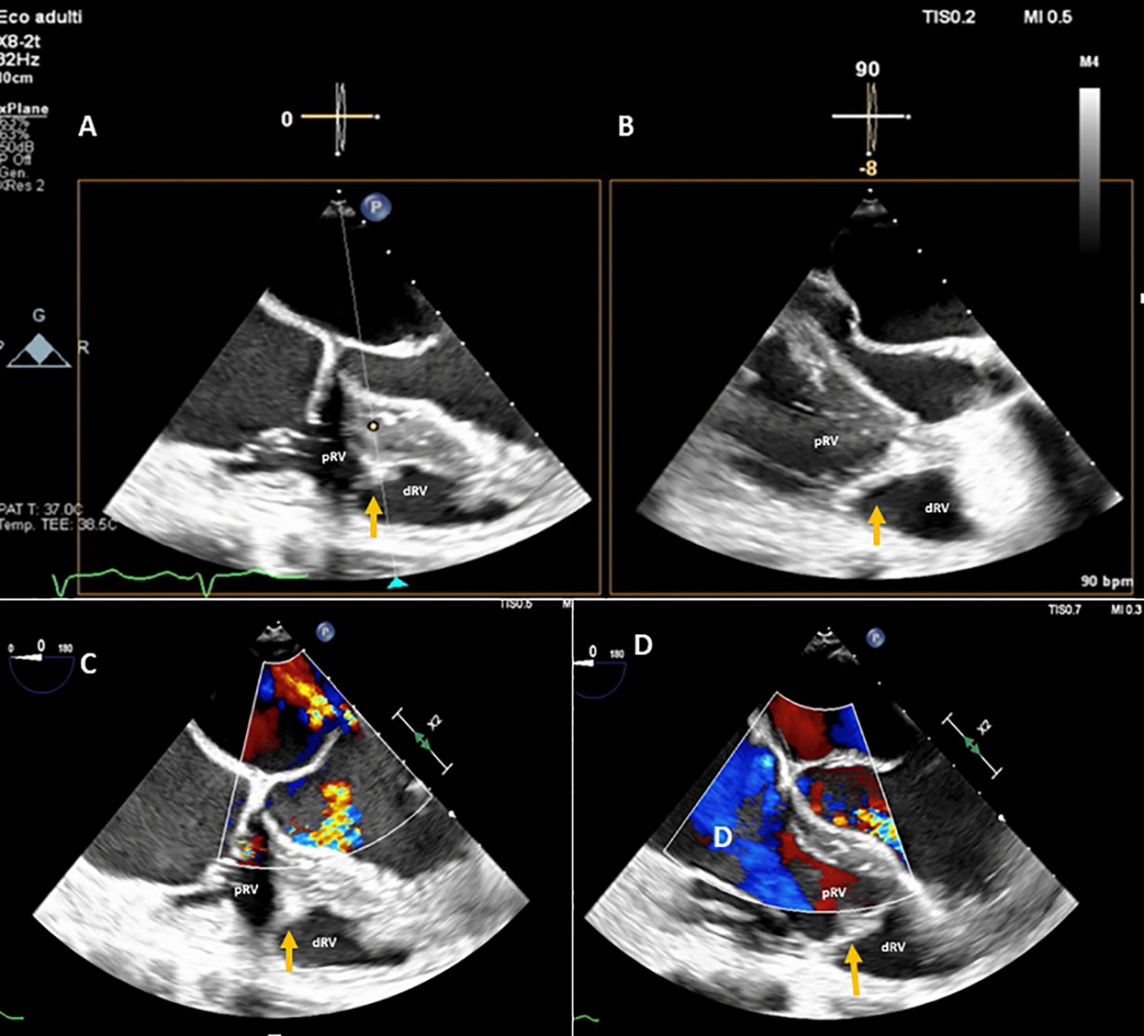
A DCRV created by an anomalous muscle band (orange arrow) diagnosed with TEE by the presence of a proximal high-pressure region under the tricuspid valve and a low-pressure distal region near the pulmonary valve outflow tract. (A,B) TEE transgastric RV inflow-outflow view (double orifice). (C,D) TEE color Doppler mid-esophageal four-chamber view focused on the RV. pRV, proximal right ventricle; dRV, distal right ventricle.
